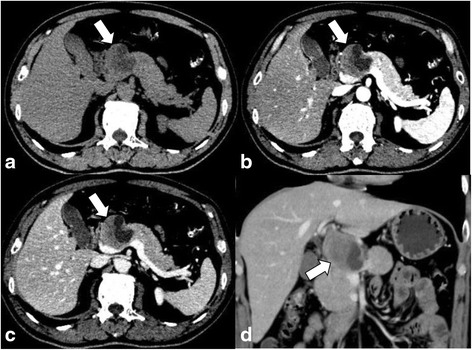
Computed tomography findings. a: An unenhanced CT scan showed a 4.8 × 4.6 cm well-defined cystic and solid mass (arrow) in the pancreatic head and adjacent to the portal vein. b: Enhanced CT scan revealed a mildly enhanced mass in the arterial phase (arrow). c: A moderately enhanced mass in the portal phase (arrow). d: A cystic and solid mass on coronal reconstruction (MPR) imaging
